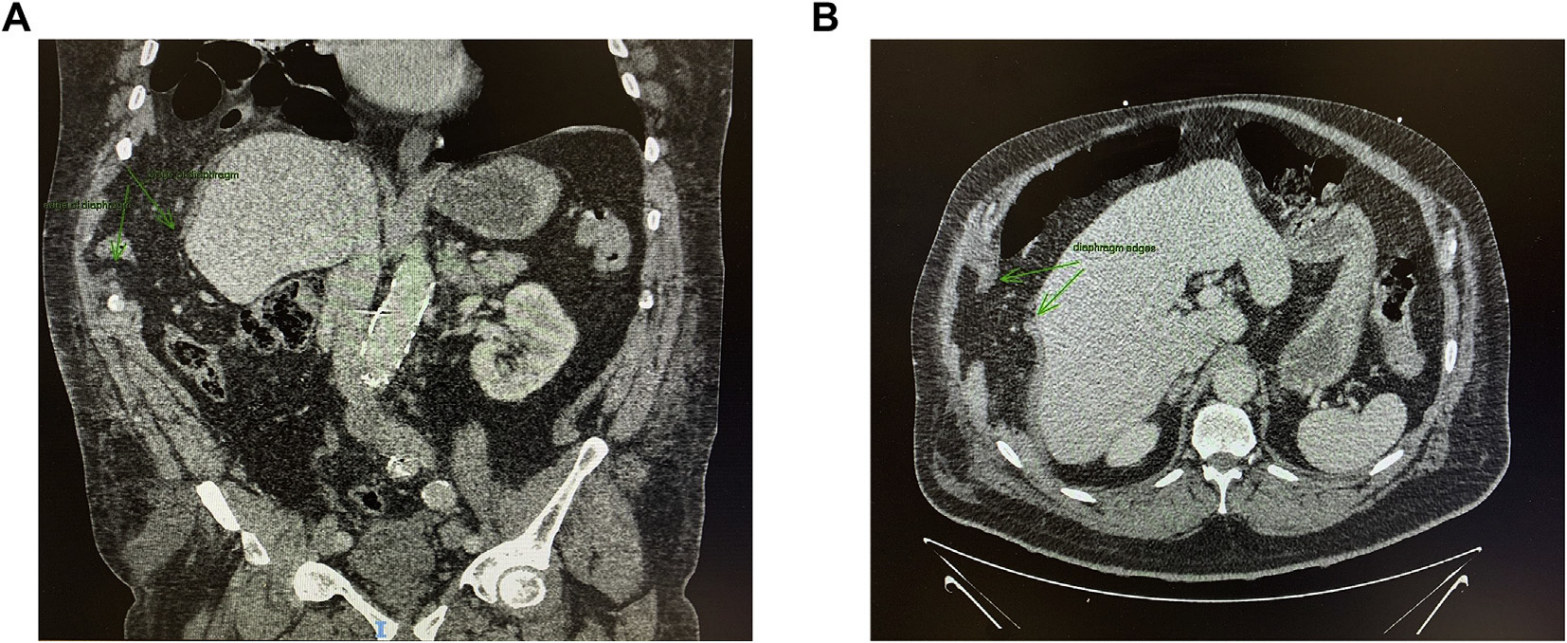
(a) (coronal view), (b) (axial view): CT scan showing diaphragmatic defect with herniation of bowel into right hemithorax.
